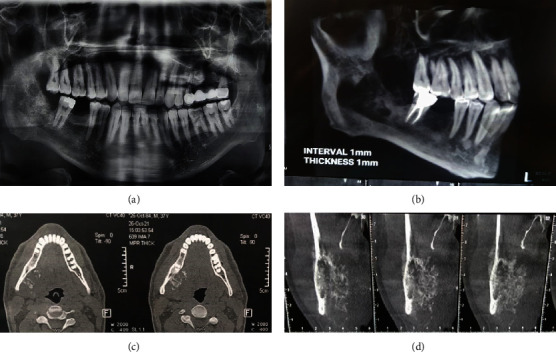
(a) Panoramic view. An ill-defined radiopaque lesion is seen on the right side of the mandible with an extension from the middle of the mandibular body to the ascending ramus. (b) 3D reconstruction. (c) An axial CT scan shows an ill-defined radiopaque mass arising from the medial aspect of the right mandibular body. (d) Coronal CT scan shows periosteal reaction on the medial and lateral aspects of the mandible, known as the Codman triangle and sunray pattern, respectively.
